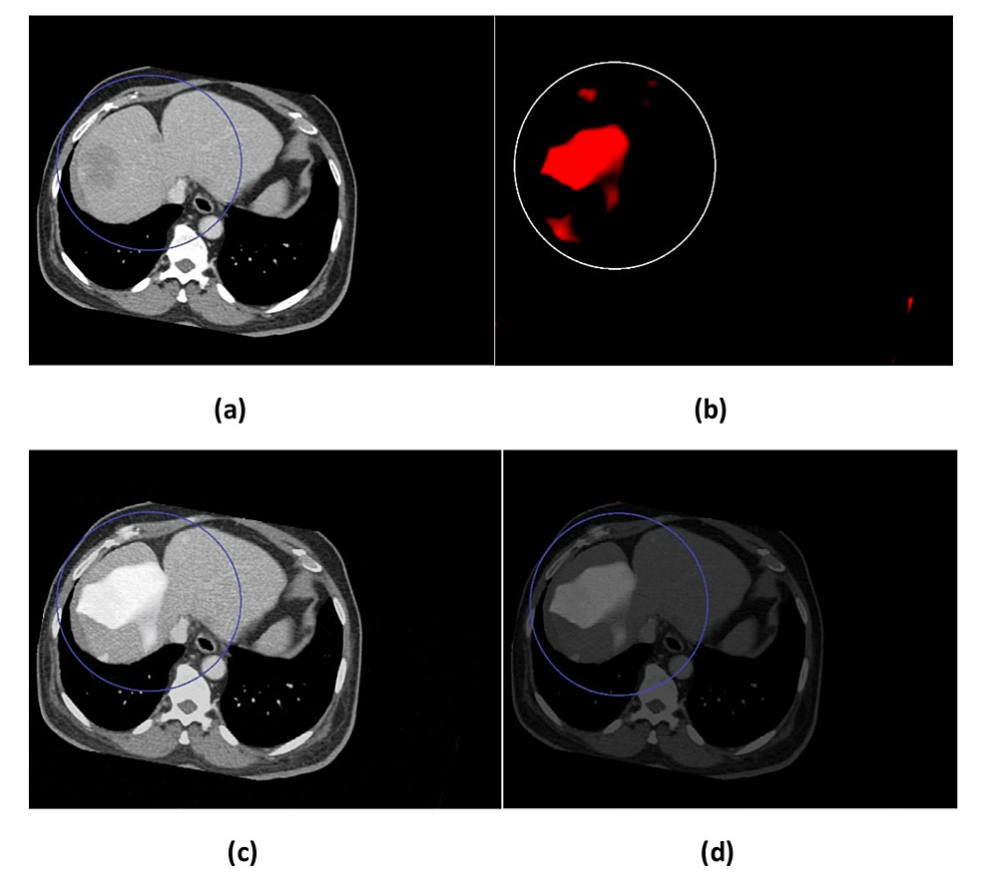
CT-MRE fusion. (a) Liver CT scan. (b) Liver MRE. (c) Bright fused image. (d) Dark fused image. CT = computed tomography; MRE = magnetic resonance elastography
